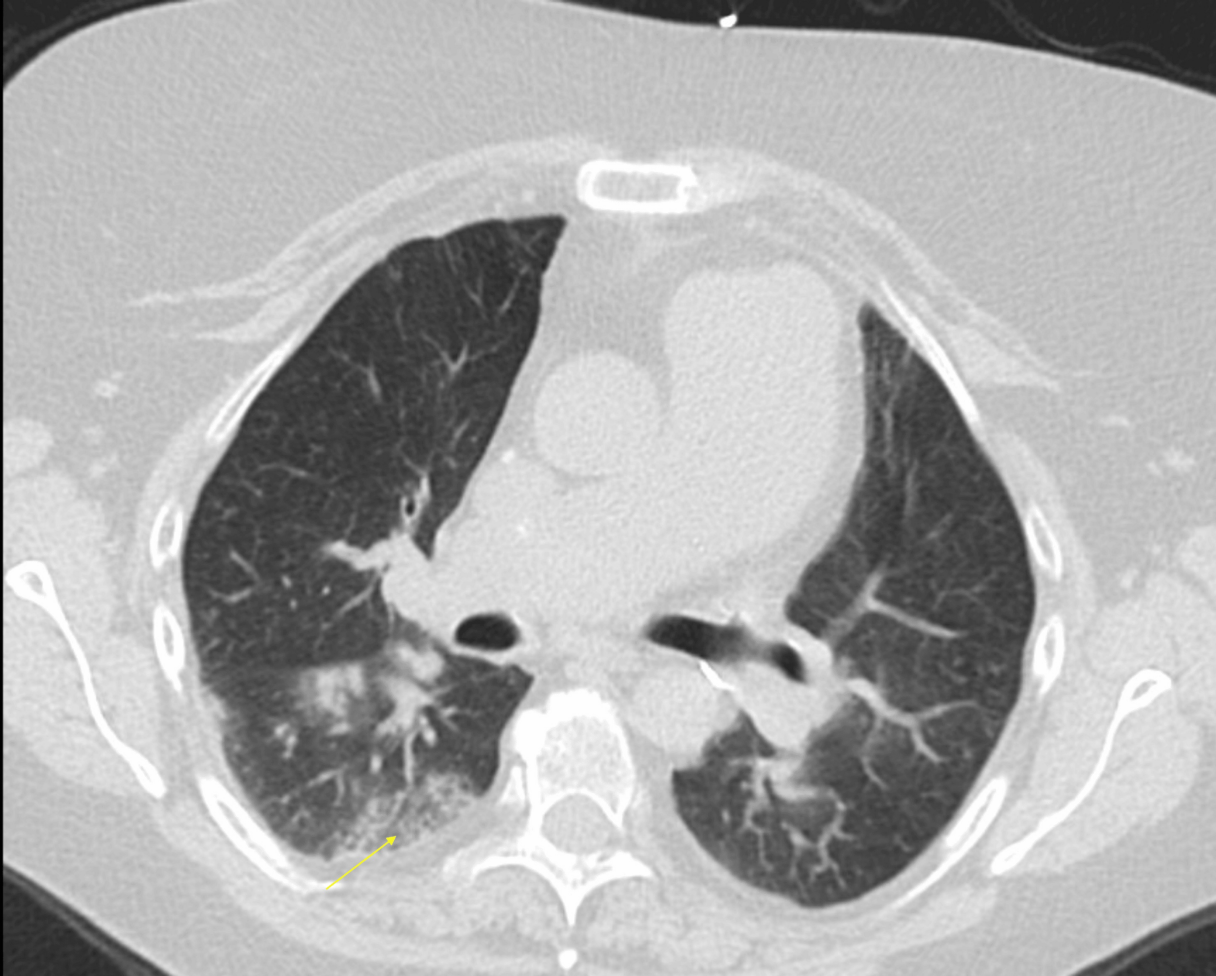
CT chest without contrast on admission demonstrating basilar air trapping consistent with possible bronchiolitis obliterans, centrilobular and subpleural glass ground-glass, and solid nodules (yellow arrow) concerning for organizing pneumonia.
